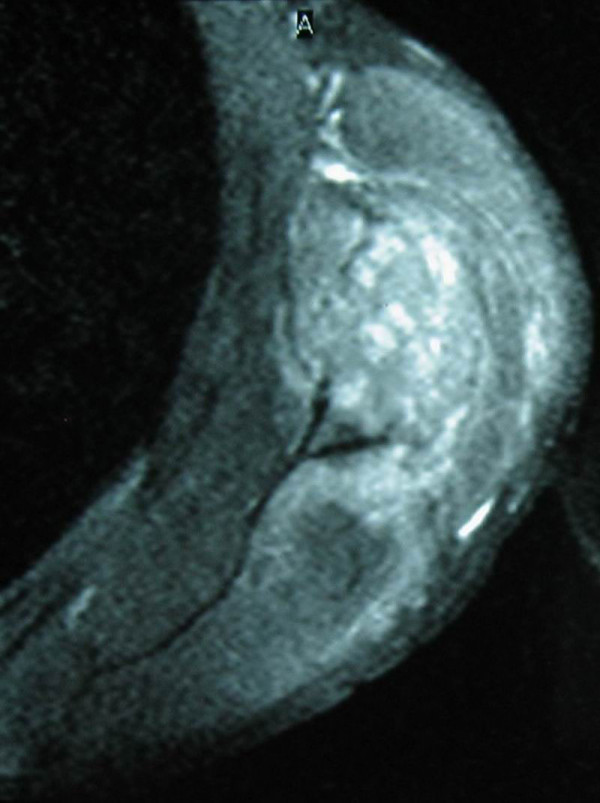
Saggital MRI image through the scapular region showed the soft tissue tumor.
